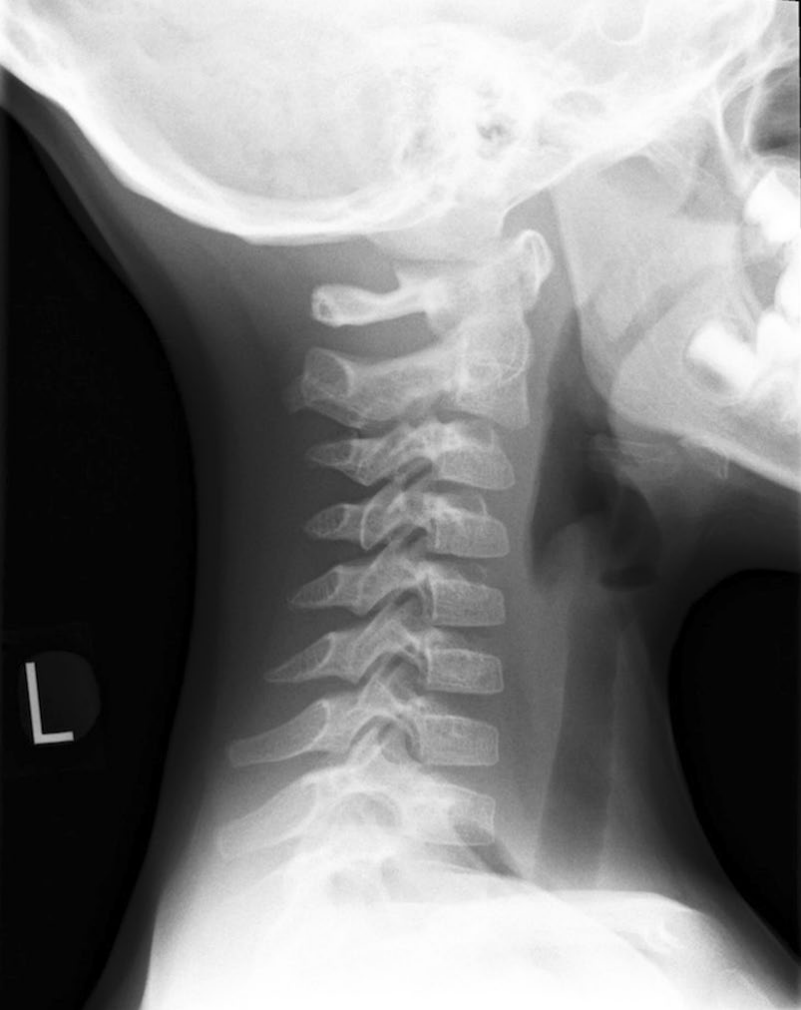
X-ray demonstrating C2 on C3 pseudosubluxation
